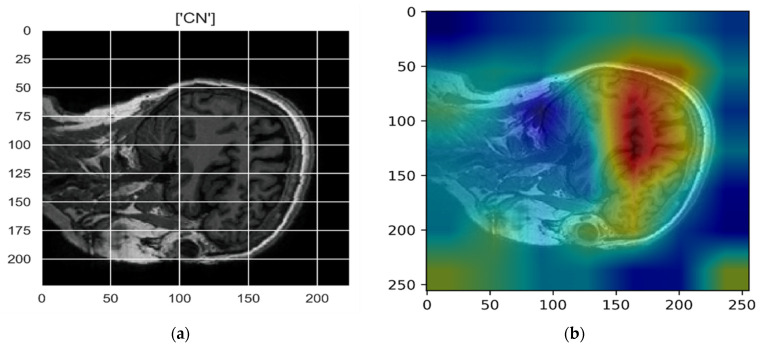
Grad-CAM explanation for the prediction of class CN: (a) MRI image, (b) Grad-CAM attention map.
